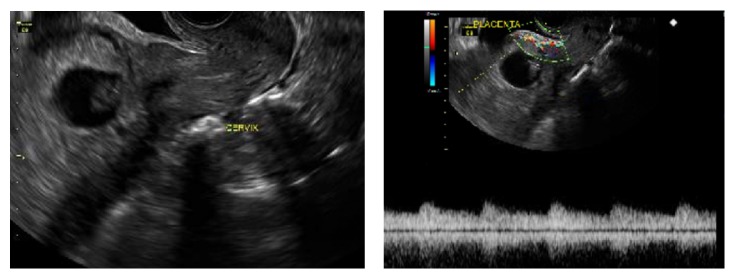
Transvaginal ultrasound showing cesarean scar ectopic pregnancy with placental circulation seen on color Doppler.
